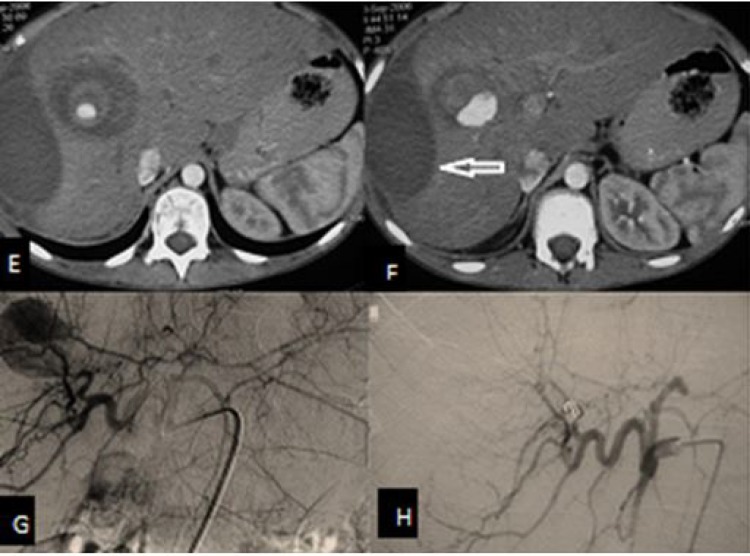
E, F) Axial contrast-enhanced CT (CECT) scan in arterial phase reveals out-pouching pseudo-aneurysm in hepatic artery branch along with intra parenchymal and sub-capsular liver hematoma (arrow). G) Selective digital subtraction angiography (DSA) from hepatic artery shows large pseudo-aneurysm in right hepatic artery. The patient underwent coil embolization for treatment of aneurysm. H) Aneurysm disappears after embolization
